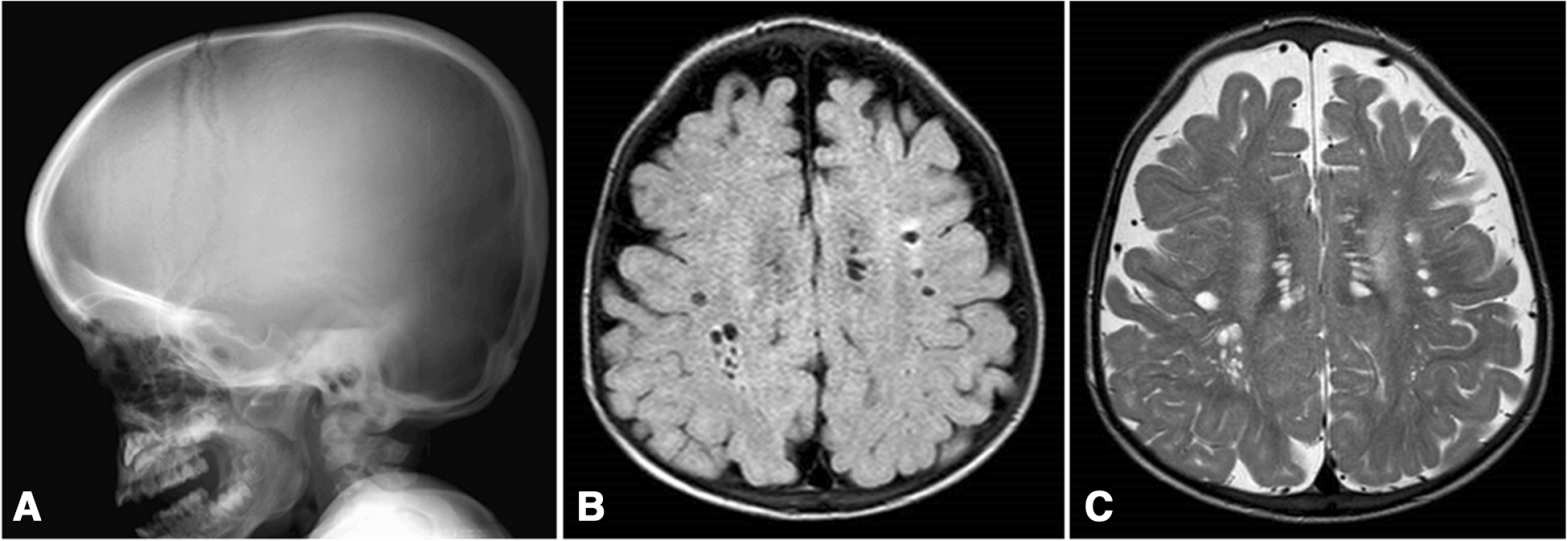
MPS IH. a Thickening of the cortical bone of the skull and abnormal “J-shaped” conformation of the sella turcica. Dilatation of the periventricular spaces in b FLAIR and c T-2 weighted magnetic resonance images of a patient aged 17 months
